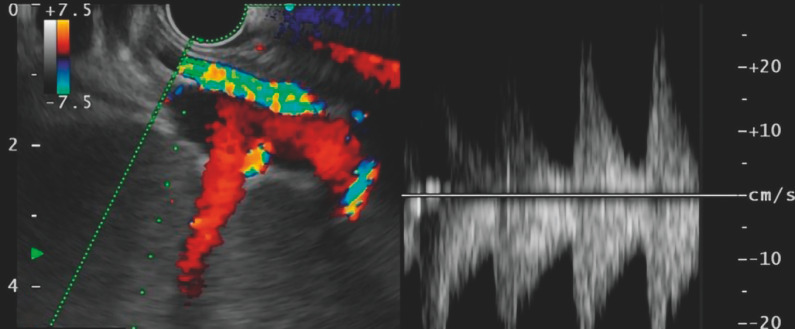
Pulsed wave Doppler image showing the peak blood flow signal at the splenic artery into the splenic hilum.
